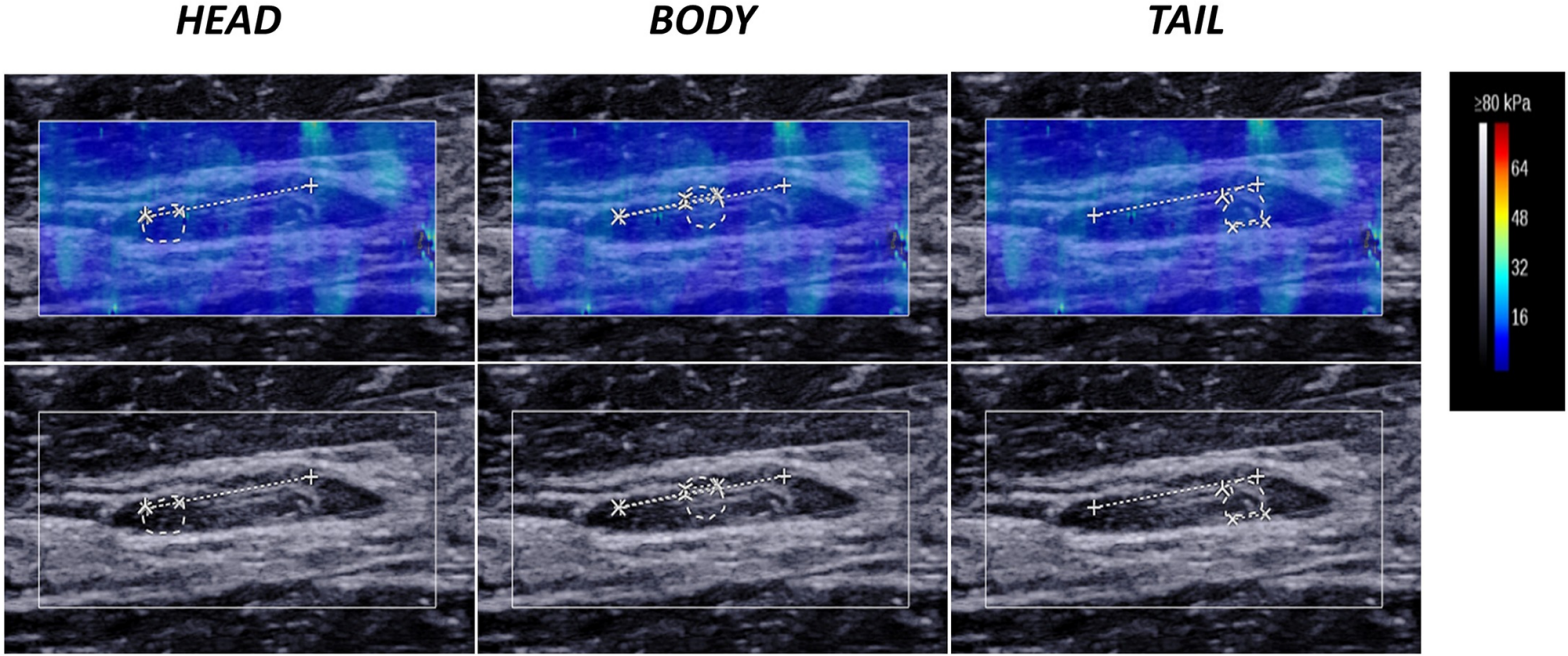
Ultrasound measurement of the thrombus on day 14 in the head, body and tail. The upper and lower row show the shear wave elastography and the gray-scale ultrasound findings of the three parts of the thrombus. The region of interest (ROI) boundary is indicated by a dashed-line circle. As shown in the colour bar, clot hardening correlates with a gradual color change within the clot from dark blue to deep red.
